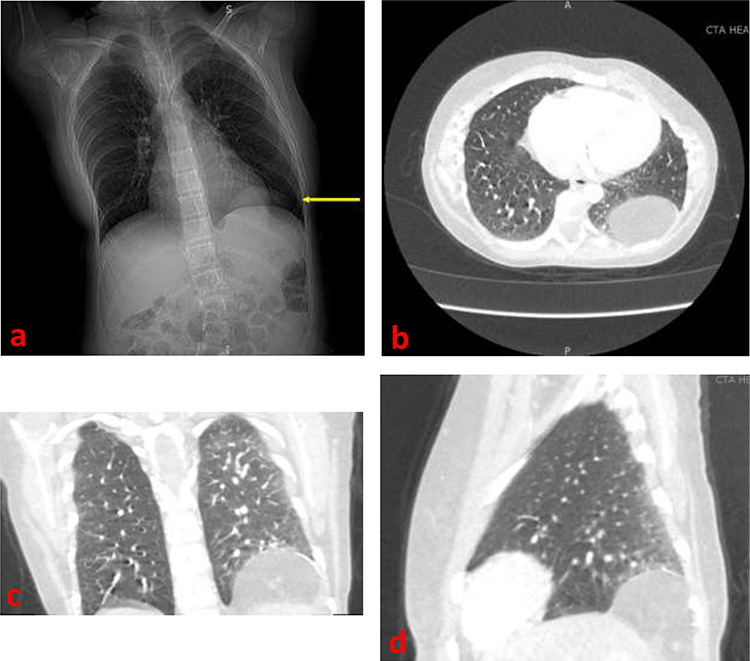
(a) Frontal chest radiograph demonstrating a left diaphragmatic hernia (arrow) (b) Axial contrast-enhanced CT image demonstrating a left diaphragmatic hernia in the left hemithorax (c) Frontal contrast-enhanced CT image demonstrating a left diaphragmatic hernia in the left hemithorax (d) Sagittal contrast-enhanced CT image demonstrating a left diaphragmatic hernia.
